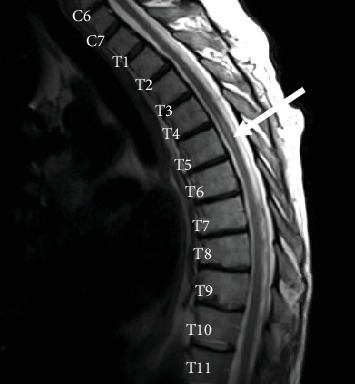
Repeat MRI with intravenous contrast of the spine demonstrating an increased T2 signal at the T5-T6 level of the spinal cord consistent with infarct.
